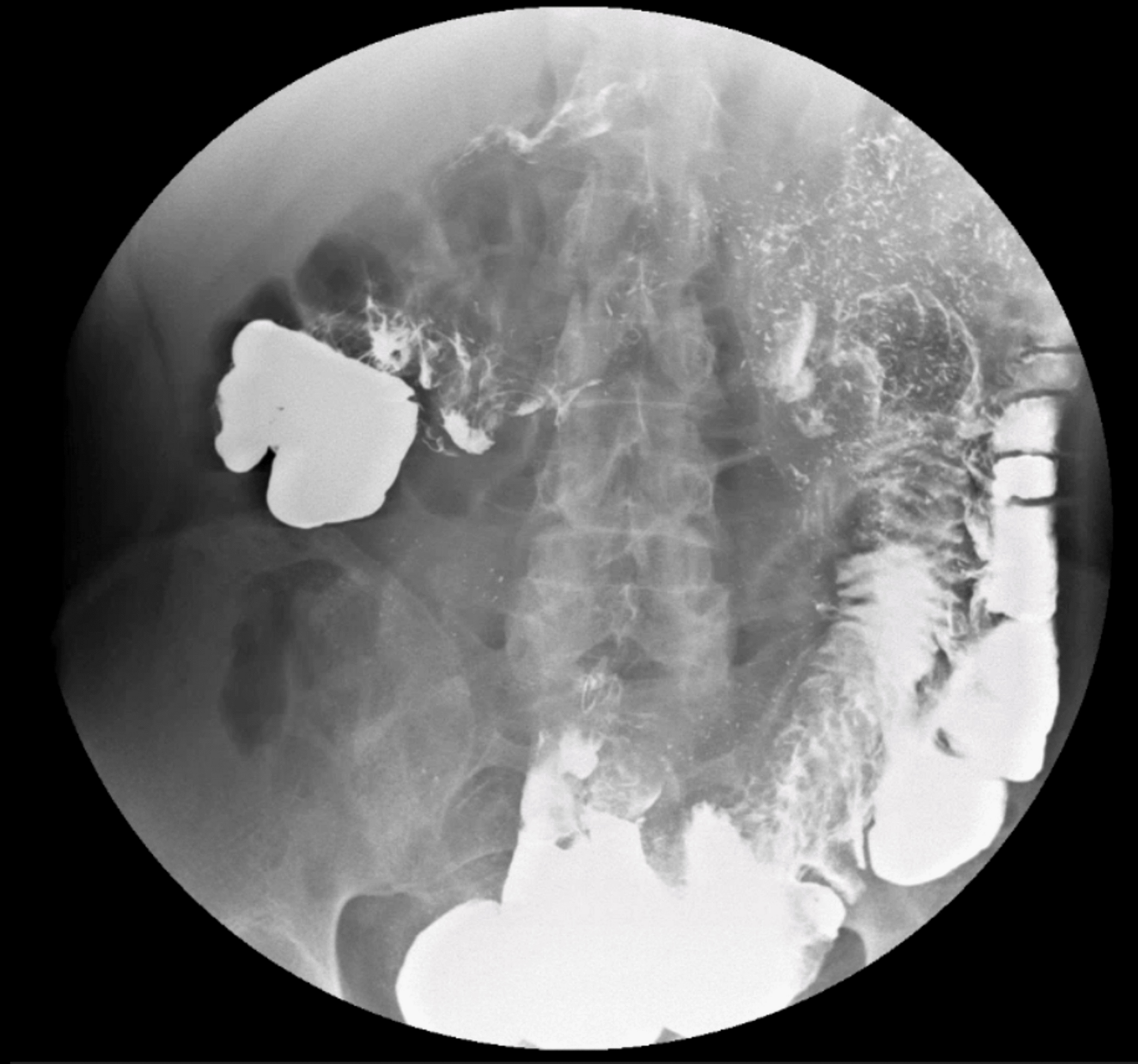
Accelerated Contrast Transit to the Distal Colon Water-soluble contrast radiograph demonstrating a rapid progression of contrast into the distal colon.
